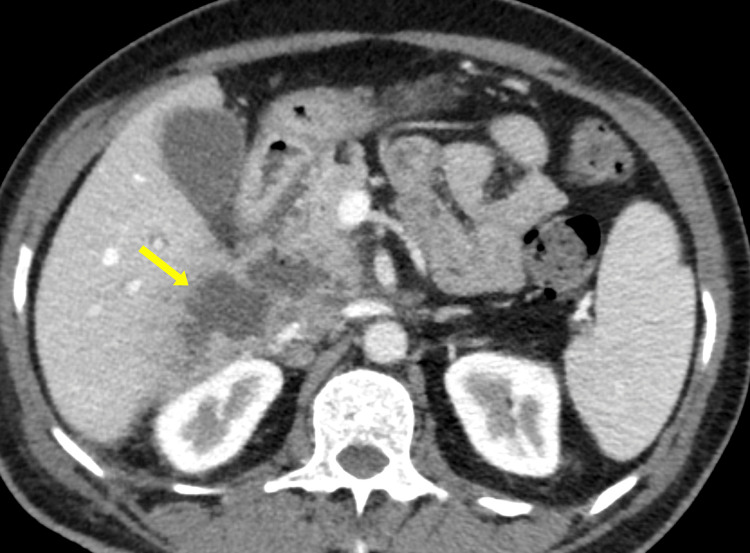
Contrast-enhanced CT scan of the abdomen (axial section) initially interpreted as a right retroperitoneal collection (yellow arrow) due to a sealed duodenal perforation.
